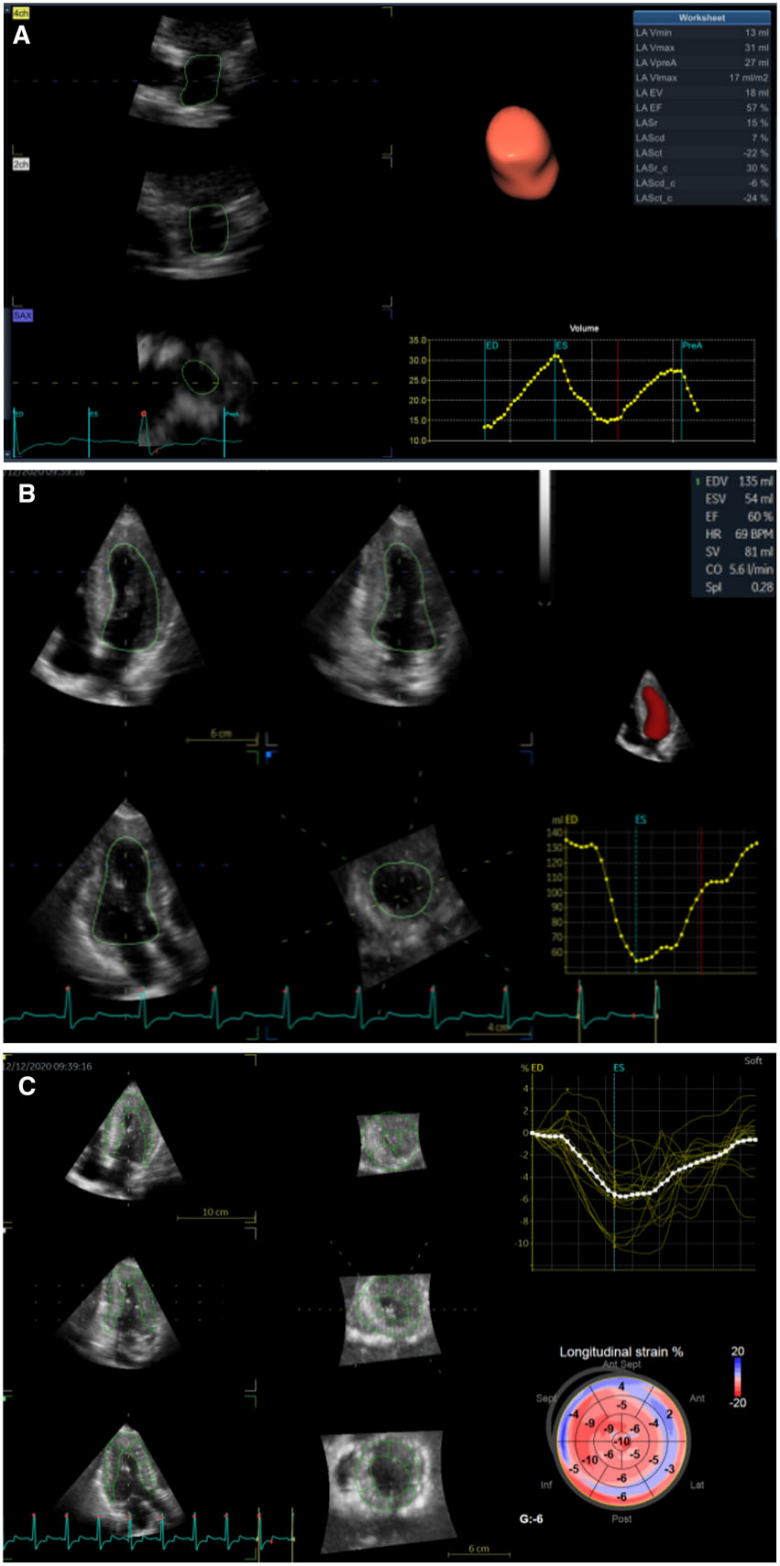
Four-dimensional echocardiography of patient 2. (A) Four-dimensional left atrial volume-strain echocardiography. (B) Four-dimensional left ventricular volume echocardiography. (C) Four-dimensional left ventricular strain.
